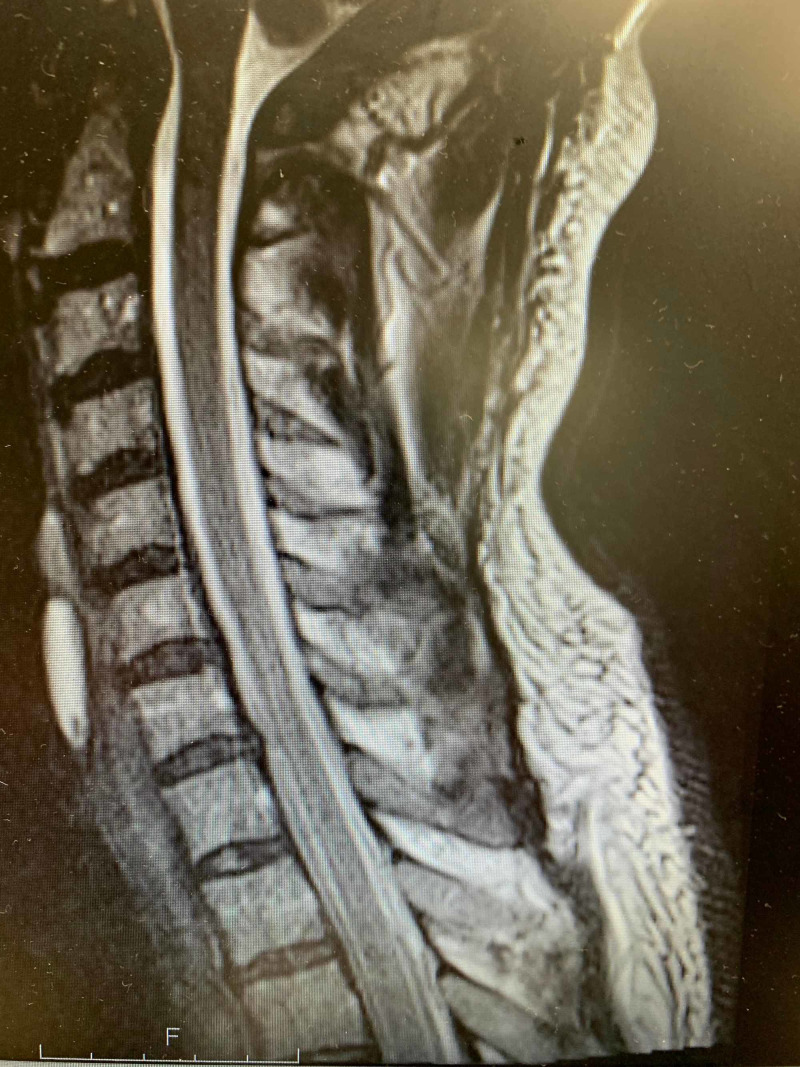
T2 weighted sagittal MRI: mild spondylosis; not enough to explain clinical findings MRI: Magnetic Resonance Imaging
